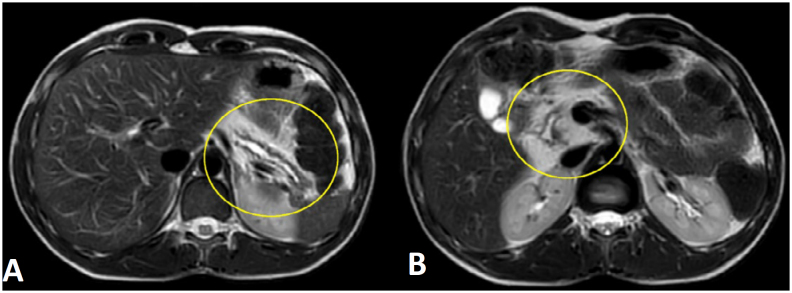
(A) T2-weighted axial image without fat signal saturation. Identification of atrophy of the pancreatic tail, ductal dilation, and homogeneous peripancreatic fat. (B) Enlargement of the head and part of the body of the pancreas by fat tissue (hyperintense).
